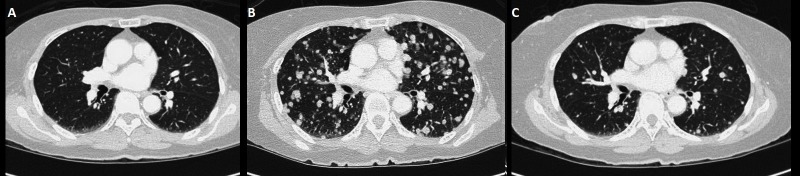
Distal response to radiation (A) Computed tomography (CT) of the chest in August 2016, showing diffuse pulmonary nodules. (B) Repeat CT of the chest in October 2016 showing remarkable interval progression of the pulmonary nodules. There was no mediastinal lymphadenopathy. (C) Follow-up CT of the chest in November 2016 following completion of palliative radiation to the neck; significant decrease in the size and number of the bilateral pulmonary nodules can be seen.
